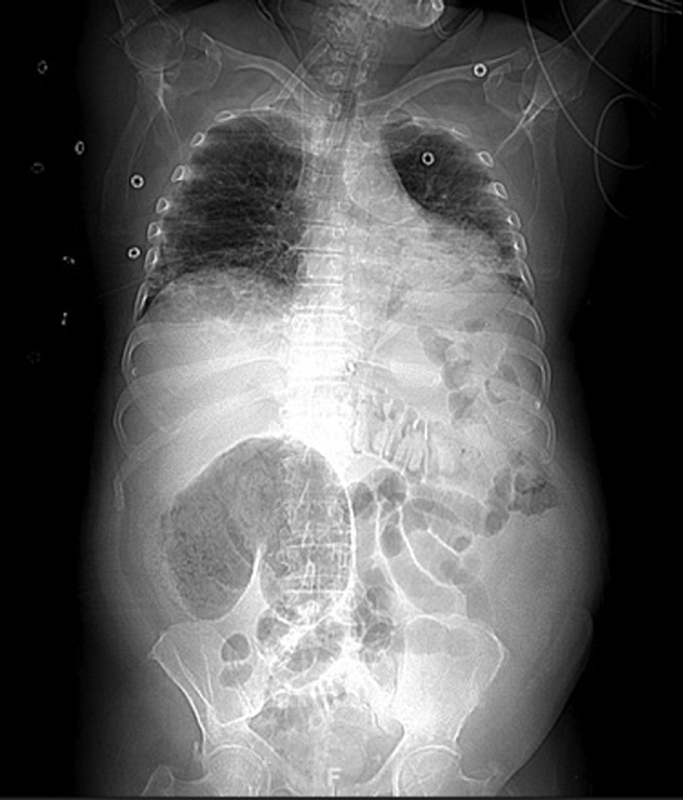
Scout view of CT abdomen/pelvis showing a distended cecum lying anterior and medial to its normal position.
